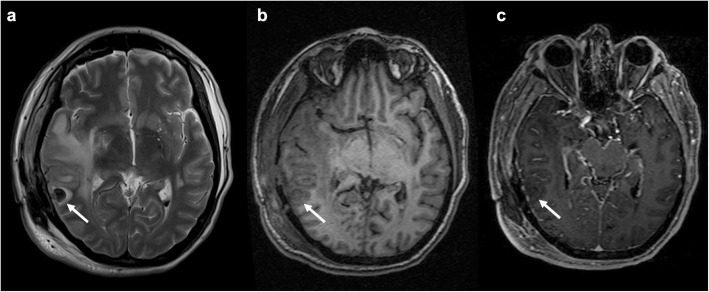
Surgical sponge. Axial T2-weighted (a), unenhanced (b), and post-contrast (c) axial T1-weighted images showing an ovoidal mass (arrow) in the right superior temporal gyrus with low signal on both sequences without enhancement
